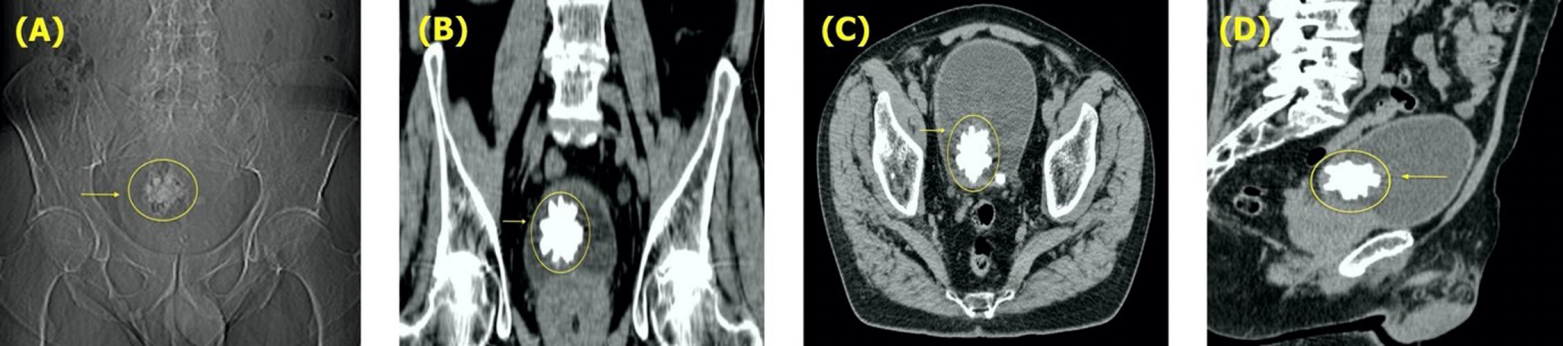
(A) Plain X-ray (KUB) demonstrating radiopaque pelvic shadow with sharp and spiculated margins (yellow arrow, and circle). Computed tomographic (CT) images. (B) Coronal, (C) Axial, and (D) Sagittal planes depicting the solitary star-shaped, spiculated Jackstone inside the bladder and intravesical protrusion of the prostate gland (yellow arrow, and circle).
